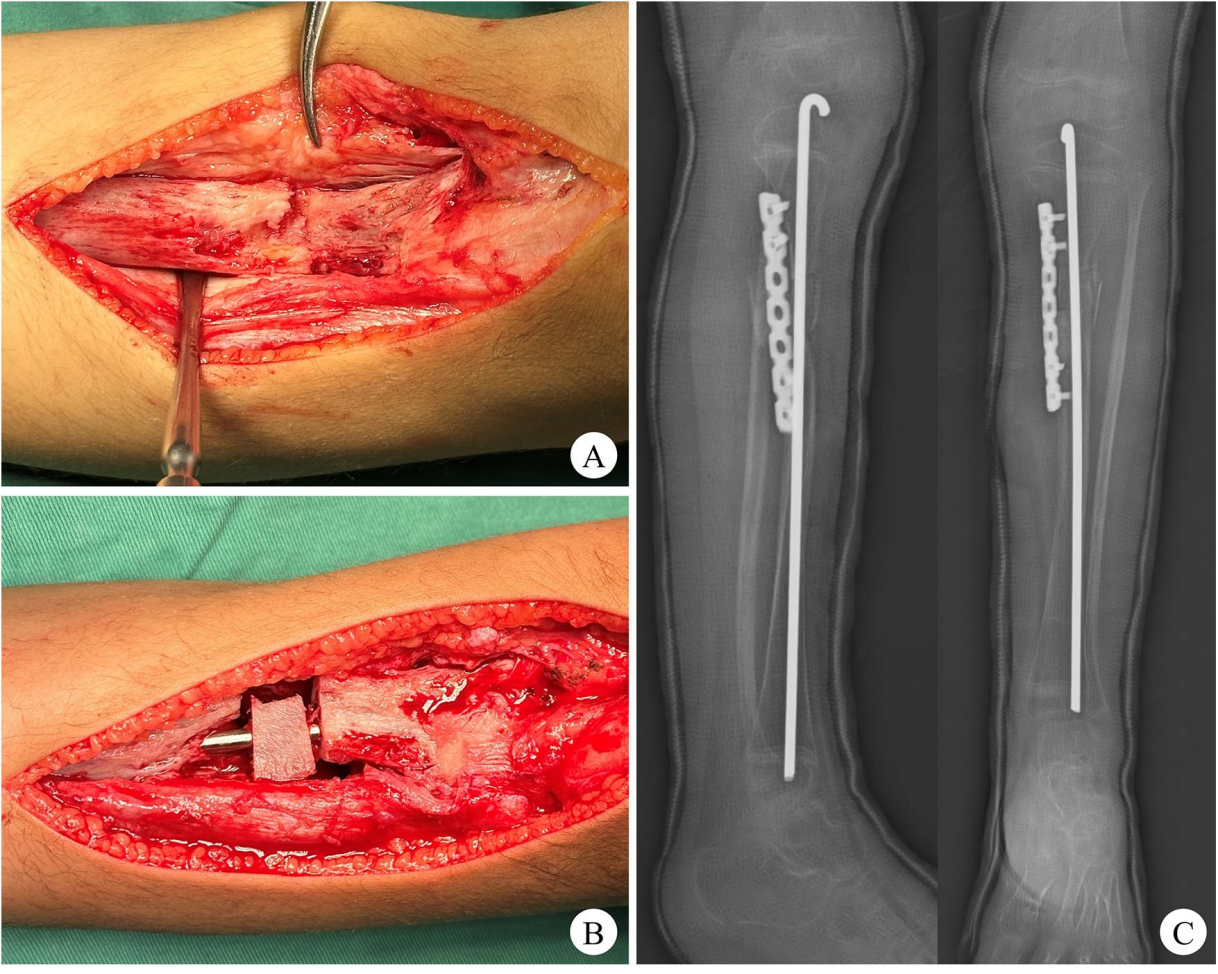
Intraoperative photographs and two-day postoperative radiographs of the tibia. (A) Localized bony dysplasia at the pseudarthrosis site with pathologically thickened fibrous periosteum. (B) The Rush rod sequentially traverses the proximal tibia, iliac bone graft, and distal tibia. (C) Satisfactory reduction (with proper alignment and position) of the osteotomy ends and optimal placement of the internal fixation.
